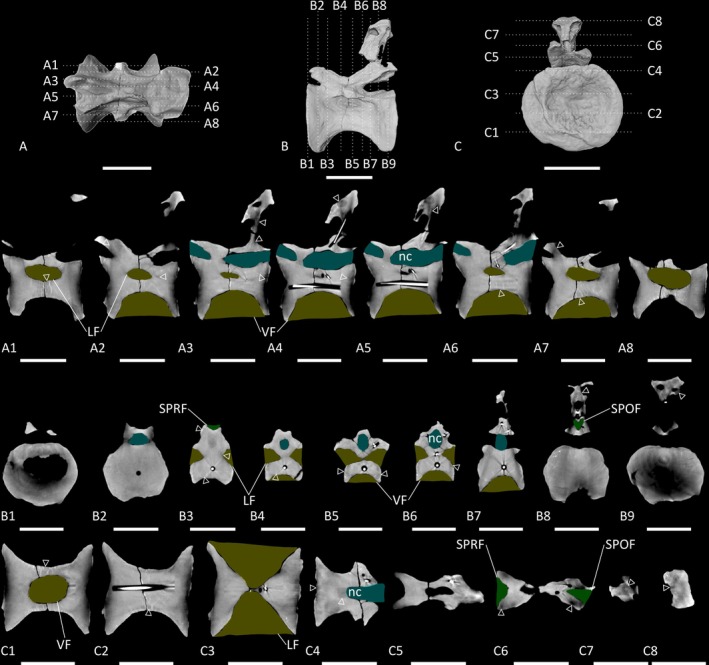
CT scan of *Tornieria africana* middle caudal vertebra MB.R.2956.6. (A–C) 3D rendering (A) dorsal view, (B) left lateral view, (C) anterior view; (A1–A8) CT scan slices in parasagittal cross‐section, (B1–B9) CT scan slices in transverse cross‐section, (C1–C8) CT scan slices in horizontal cross‐section. LF, lateral fossa; nc, neural canal; SPOF, spinopostzygapophyseal fossa; SPRF, spinoprezygapophyseal fossa; VF, ventral fossa. Key: Blue highlight, neural canal; green highlight, neural spine fossa; yellow highlight, centrum fossa; white arrow, pneumatic bone texture. Scale bars = 100 mm.
